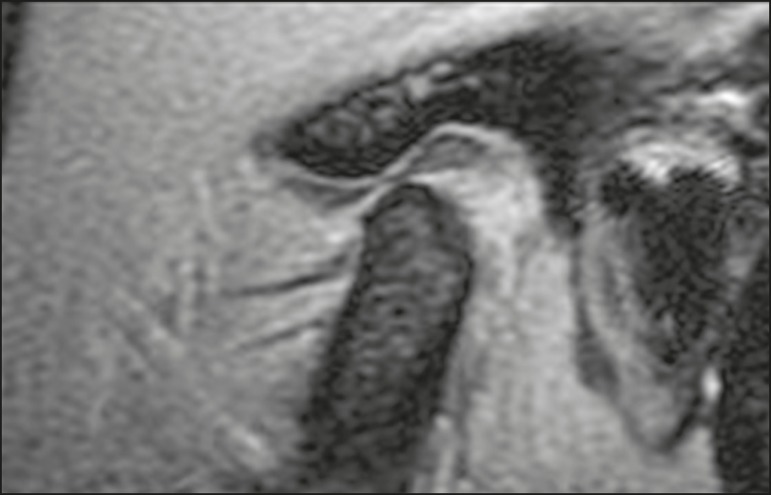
T1-weighted central sagittal MRI scan of the TMJ. In the closedmouth
